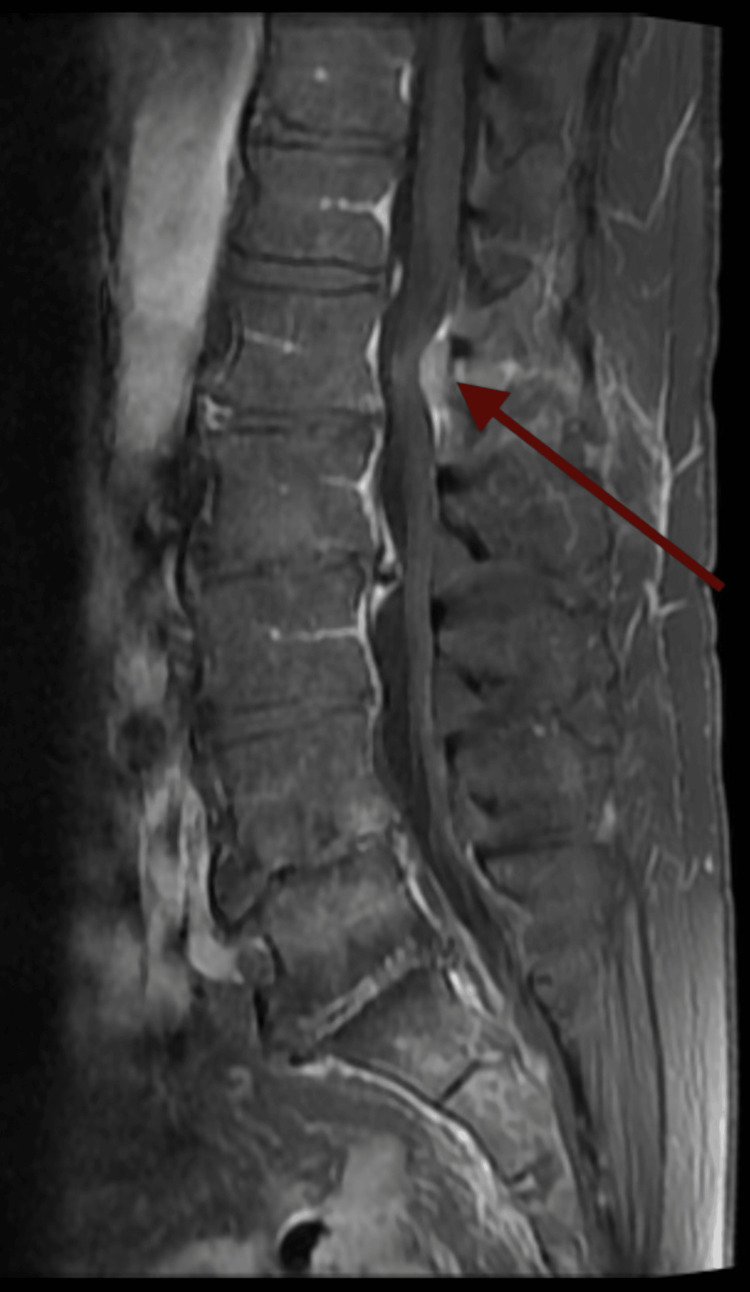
MRI demonstrating intramedullary spinal cord lesion at the level of L2 The red arrow depicts a metastatic lesion infiltrating the intramedullary space. MRI: Magnetic resonance imaging
